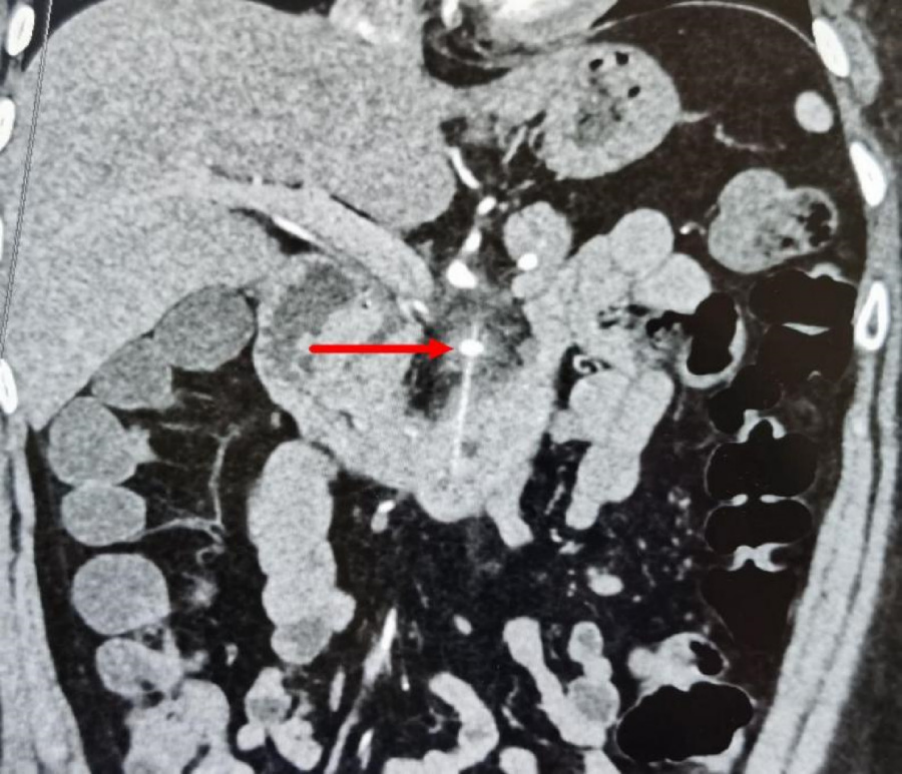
CT reconstruction a linear high-density in the horizontal segment of the duodenum.
